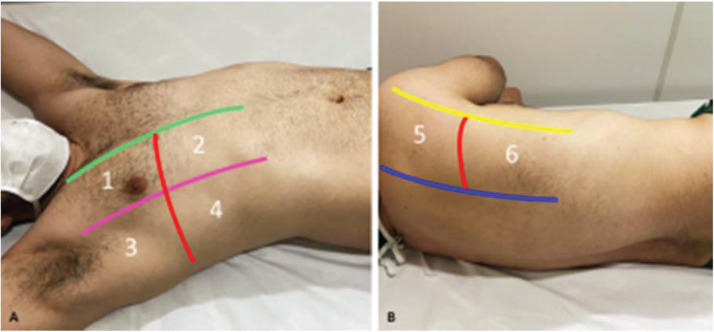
Transducer sites for lung ultrasound. The six sites are anatomically divided by the parasternal line (green line), anterior axillary line (pink line), posterior axillary line (yellow line), and paravertebral line. The subdivision in the superior and inferior takes into account the well-established Bedside Lung Ultrasound in Emergency (BLUE) protocol.
